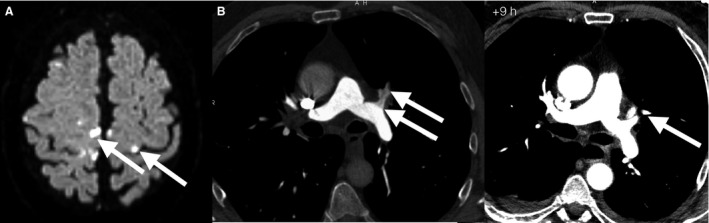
(A) Magnetic resonance imaging scan of the head (diffusion‐weighted imaging) multiple cerebral thromboembolisms according to the central region, superior, and middle frontal gyrus. (B) CT scan of the chest 6 h after the first symptoms: multiple pulmonary thromboembolisms of the segmental arteries. Follow‐up CT scans of the chest 9 h later: no pulmonary thromboembolism of the same segmental arteries. CT, computed tomography; DWI, diffusion‐weighted imaging.
